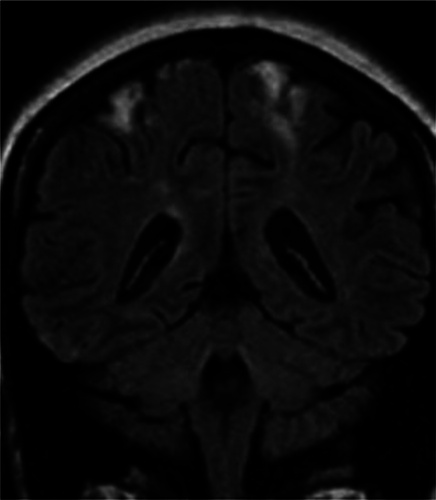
Plain MRI of the brain showing bilateral watershed infarcts between bilateral anterior cerebral artery and middle cerebral artery territories.
